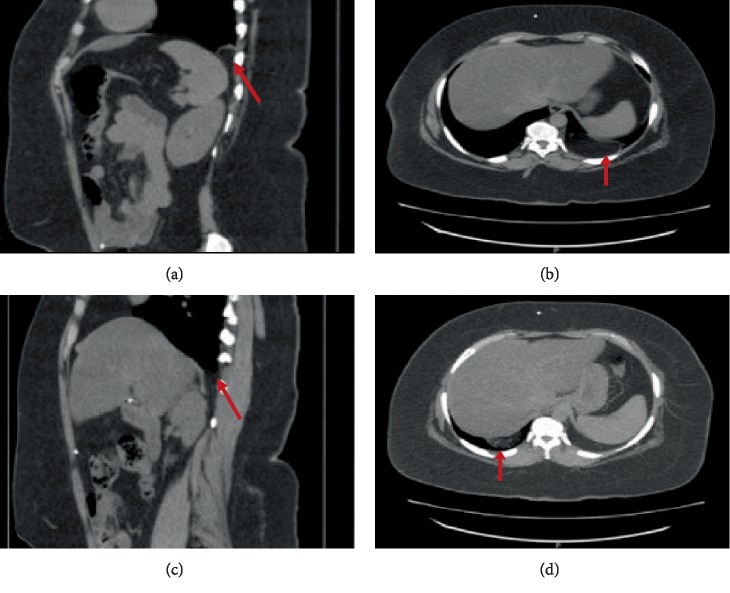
Computed Tomography (CT) scan of the abdomen showing a 1.6 cm left-sided Bochdalek Hernia in a sagittal (a) and axial (b) plane containing fat (red arrow) and a 1.5 cm right-sided Bochdalek Hernia in a sagittal (c) and axial (d) plane containing only fat (red arrow).
